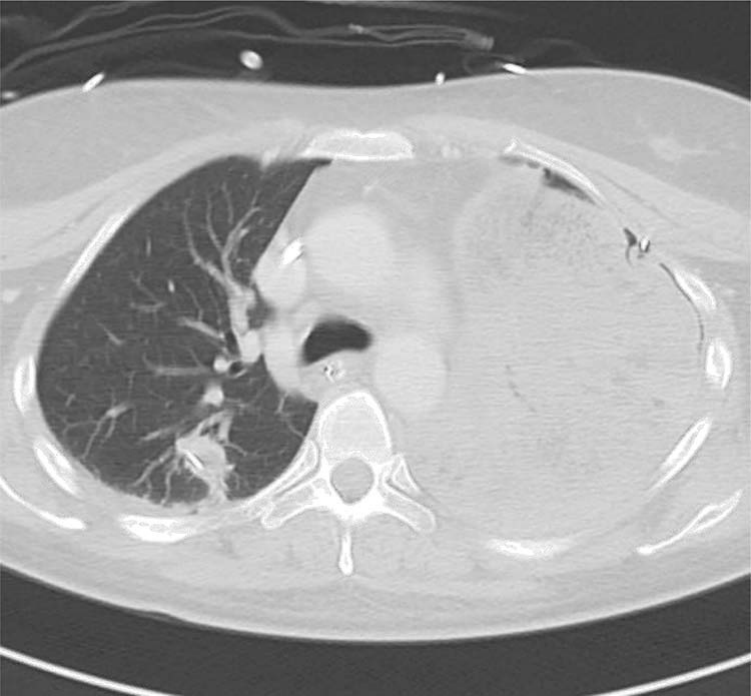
Computed tomography images of 2 days after initial emergency operation.
